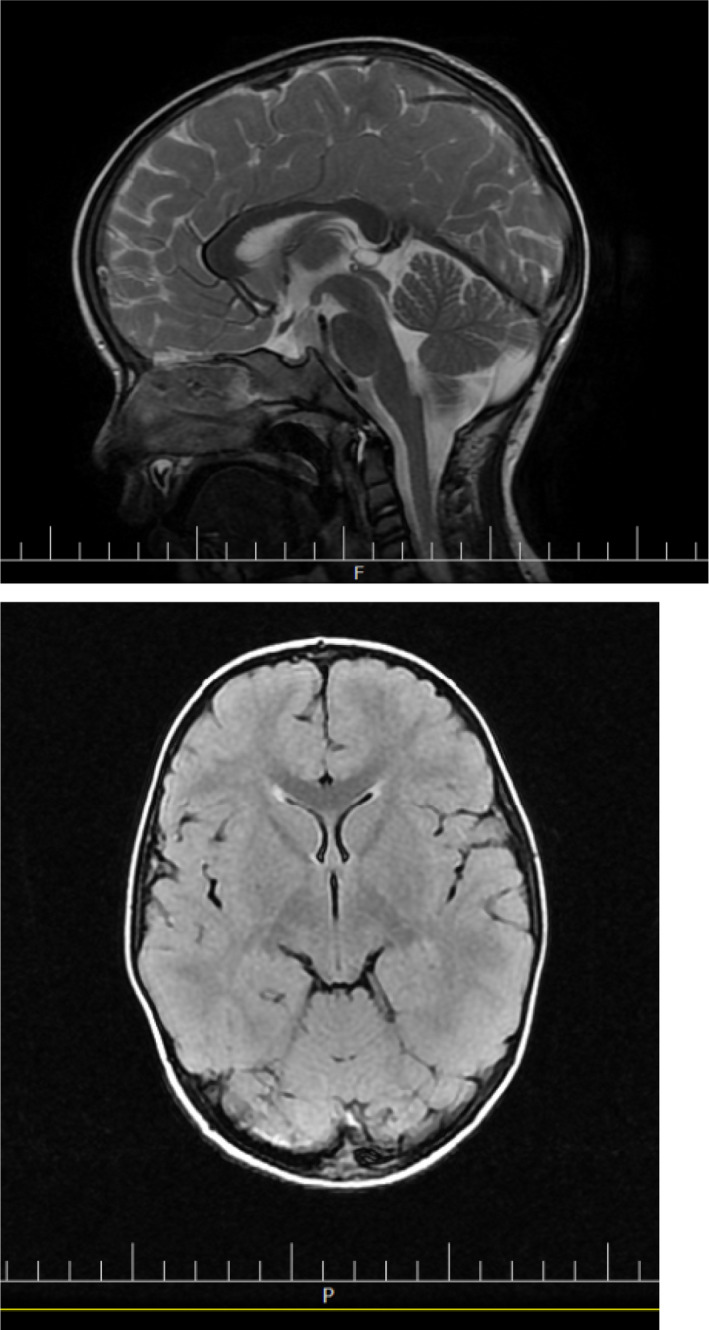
Brain MRI in a subject with ataxia‐telangiectasia when aged 2 years old
